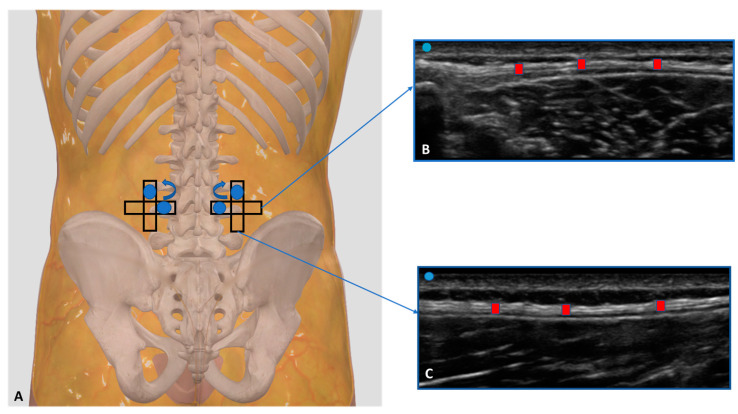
(A) Ultrasound measurements protocol of TLF thickness at the L3 level of the lumbar spine. Starting from the transversal axis to the long axis and rotating the probe 90° degree. Probe: black rectangle. (B) Transversal/short axis; (C) longitudinal/long axis. Red rectangle: TLF thickness.
